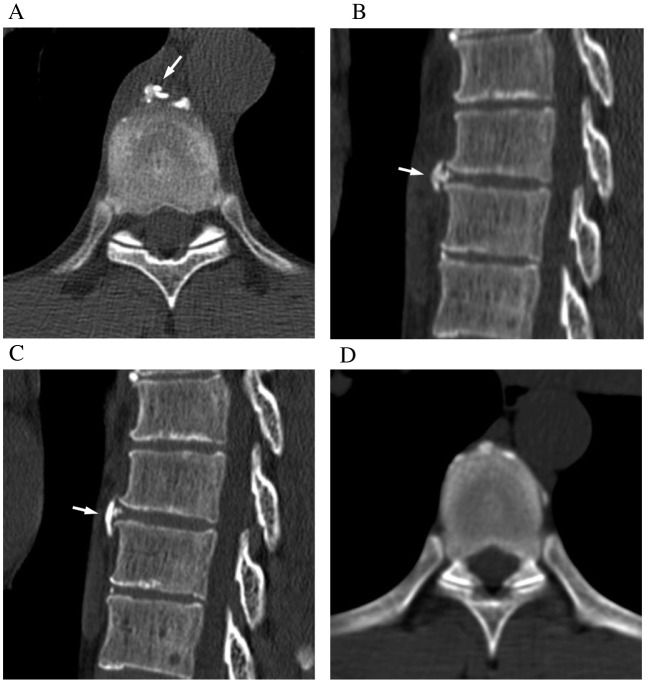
A 35-year-old man with back pain and epigastric pain (Patient #9). A Axial CT images with bone window setting shows the paravertebral calcification in the anterior aspect vertebrae at the level of Th 8/9 intervertebral disc. B Sagittal CT image reveals an elongated crescent-shaped calcification adjacent to the calcification of the annulus fibrosus (arrow). The paravertebral calcification demonstrates lower attenuation than the calcification of the cortical bone of the vertebral arch. C Sagittal CT image obtained 1 month later reveals a craniocaudal expansion of the paravertebral calcification (arrow). D CT scan obtained 4 years prior shows no evidence of paravertebral calcification
